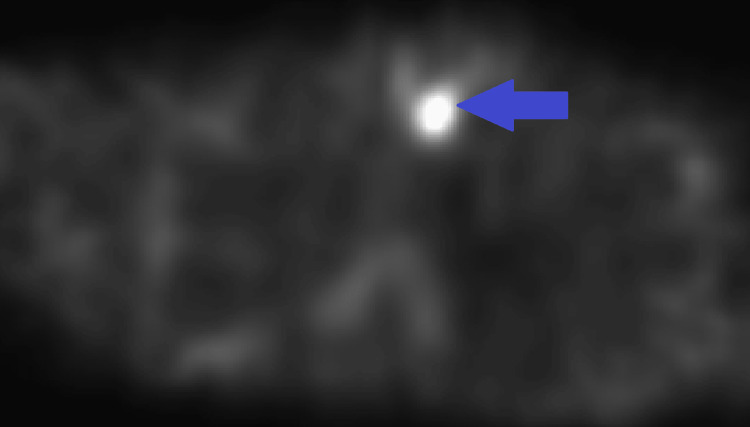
Single photon emission computed tomography (SPECT) images from Tc-99m sestamibi parathyroid nuclear medicine scan, with focal radiotracer uptake corresponding to an anterior mediastinal nodule (blue arrow) confirming the diagnosis of parathyroid hyperplasia.
